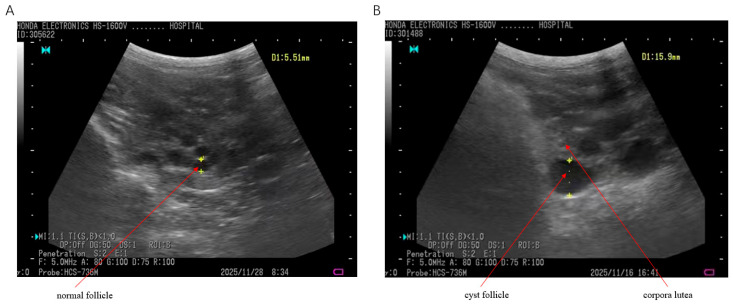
Ultrasonographic morphology of ovarian tissue in gilts; the arrows point to follicles and corpora lutea (The corpus luteum features solid parenchymal tissue inside and manifests as a regular circular gray area under ovarian ultrasonography); The yellow-marked regions in Figure 2A represent normally developing follicles, while those in Figure 2B represent cystic follicles. (A) Normal ovary; (B) Follicular cyst ovary.
